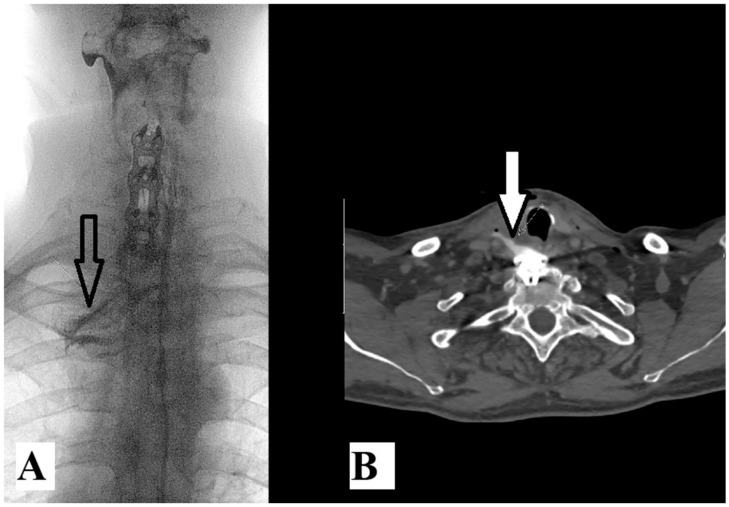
Fluoroscopy image of contrast media swallow test (A) reveals extralumination (arrow) of water-soluble contrast media through the perforated upper esophagus. Axial CT image (B) at the level of the first thoracic vertebral body showing extralumination (arrow) of previously swallowed water-soluble contrast media into the prevertebral and carotid space of the neck.
